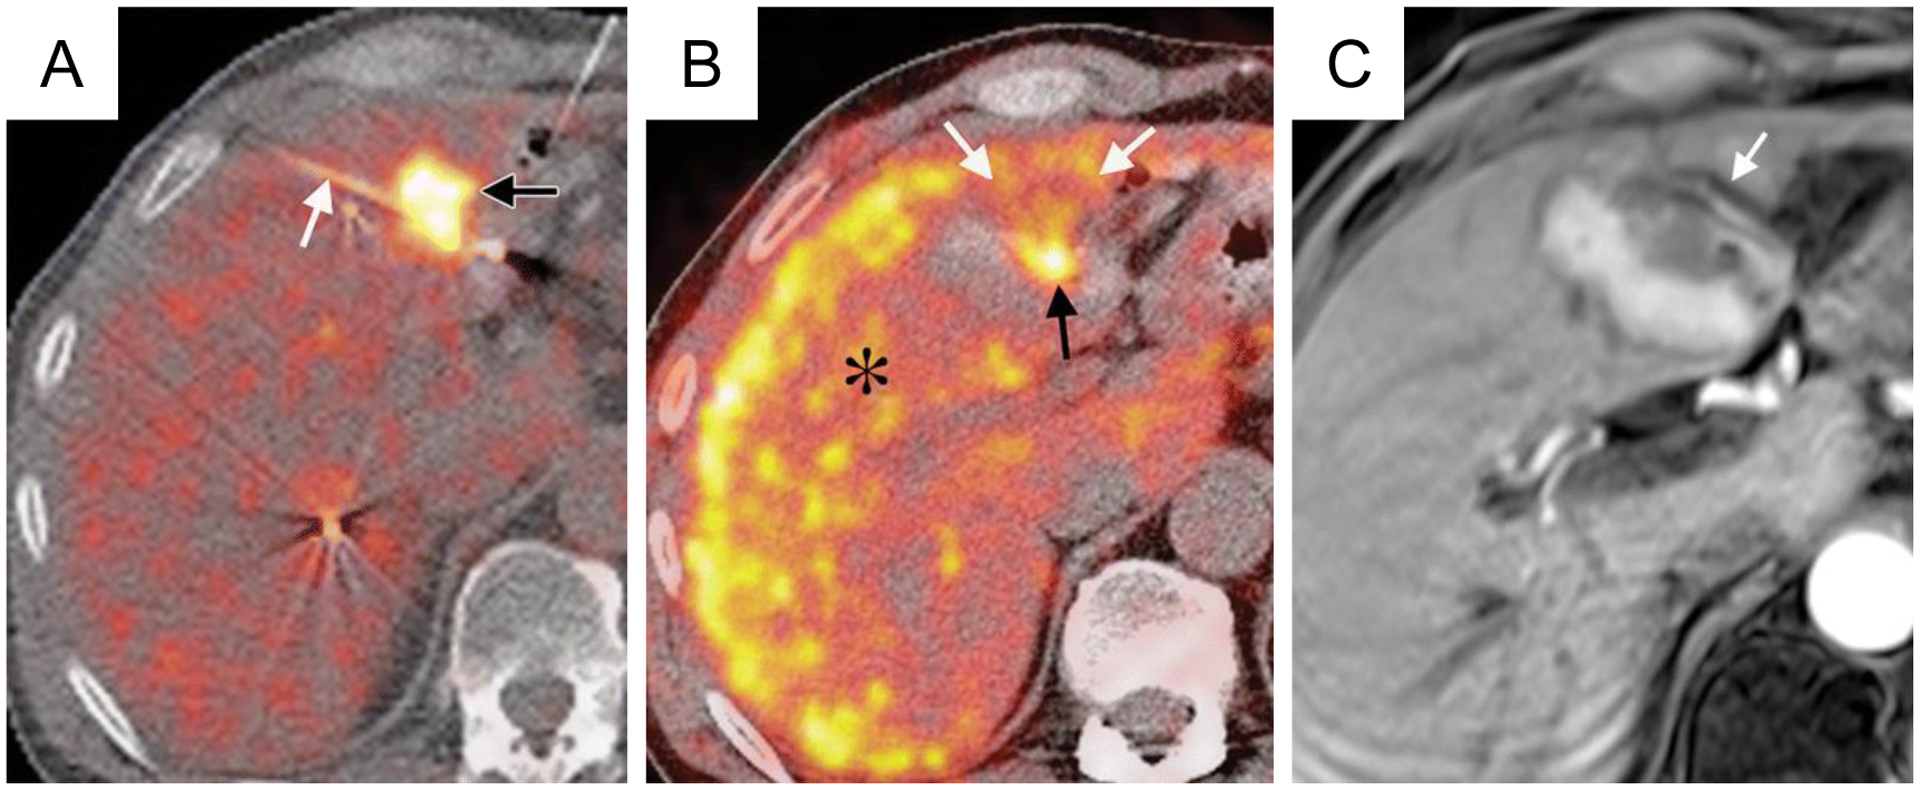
Pre and intraprocedural combined ^18^F-FDG and ^13^N-ammonia PET during microwave ablation compared to contrast-enhanced T1w MRI postprocedure. (a) Fluorodeoxyglucose (FDG) PET/CT image obtained at single breath hold shows FDG uptake in the metastasis (black arrow) and the microwave ablation probe (white arrow) positioned in the posterior half of the tumor. (b) Intraprocedural perfusion PET shown as a fused PET/CT image, with PET image in color, was obtained immediately after microwave ablation. FDG administered before the procedure remains trapped within the tumor (black arrow). Healthy, unablated liver activity (*) is increased by ^13^N-ammonia perfusion. A photopenic ablation margin partially surrounds the ablated tumor from 2 o’clock to 10 o’clock; however, the margin is incomplete anteriorly from 10 o’clock to 2 o’clock (white arrows). (c) Contrast-enhanced T1-weighted MR image acquired 24 hours after the procedure (1.5-T imager; volumetric interpolated brain examination; 4.1/1.6; flip angle, 10°) demonstrates an unsuspected blood vessel (arrow) along the anterior aspect of the ablated tumor; this vascular heat sink likely contributed to the inadequate ablation margin anteriorly. Figure adapted from [22].
